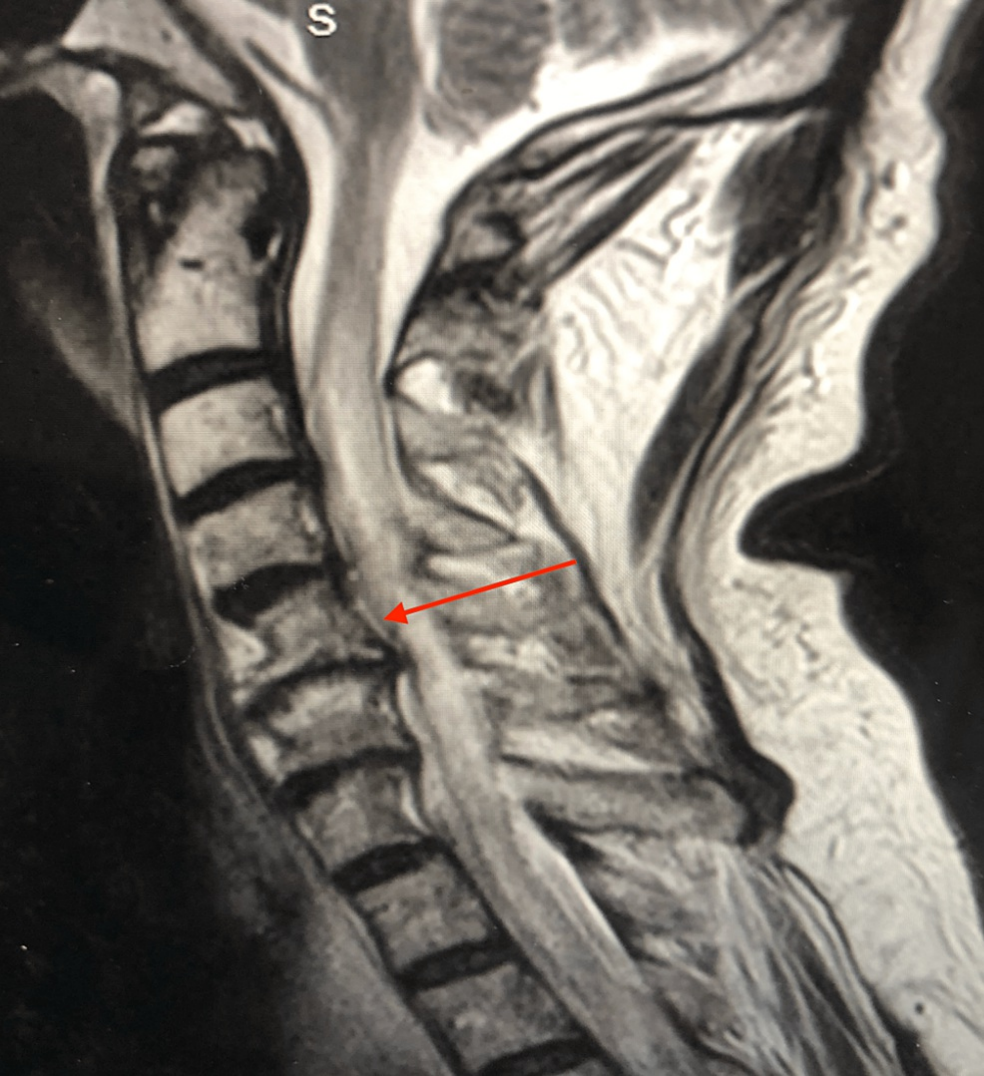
MRI with contrast of the cervical spine showing intraspinal metastasis
